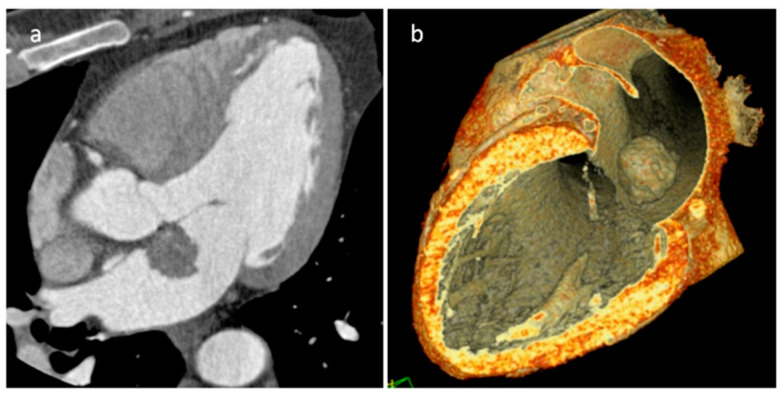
Left atrial myxoma on three-chambers view (a) and three-dimensional volume rendering (b) CT images in a 45-year-old male patient.
